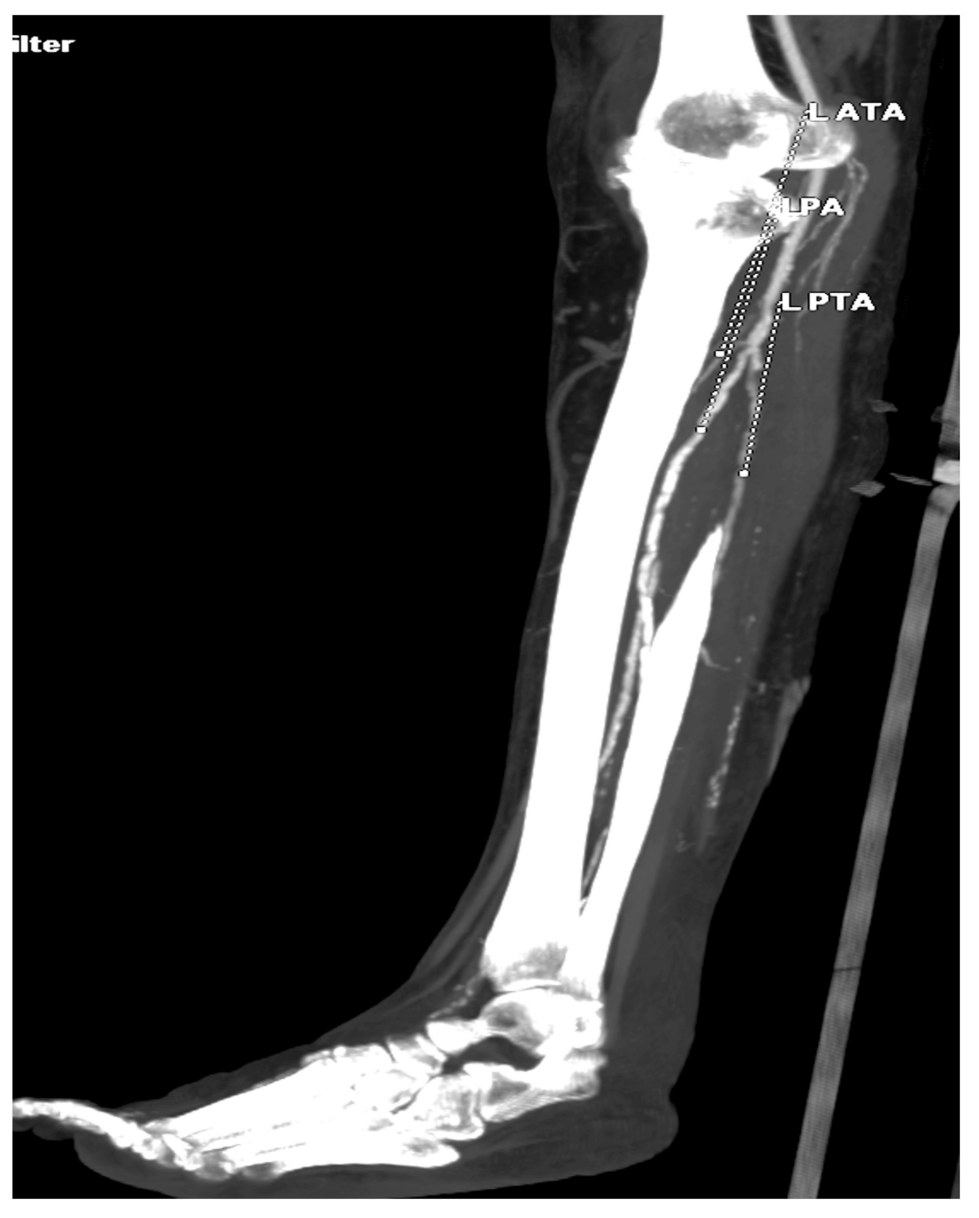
Features of peripheral arterial disease in a CT arteriogram of the lower limb vessels.
